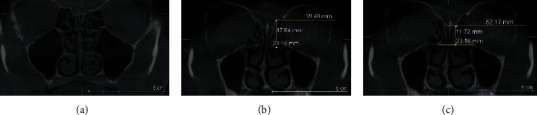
(a) Coronal plan of CBCT demonstrating type 1 TMS classification on the left side. (b) OFL-ER measurement and (c) OFL-CP measurement coronal plan of CBCT.
